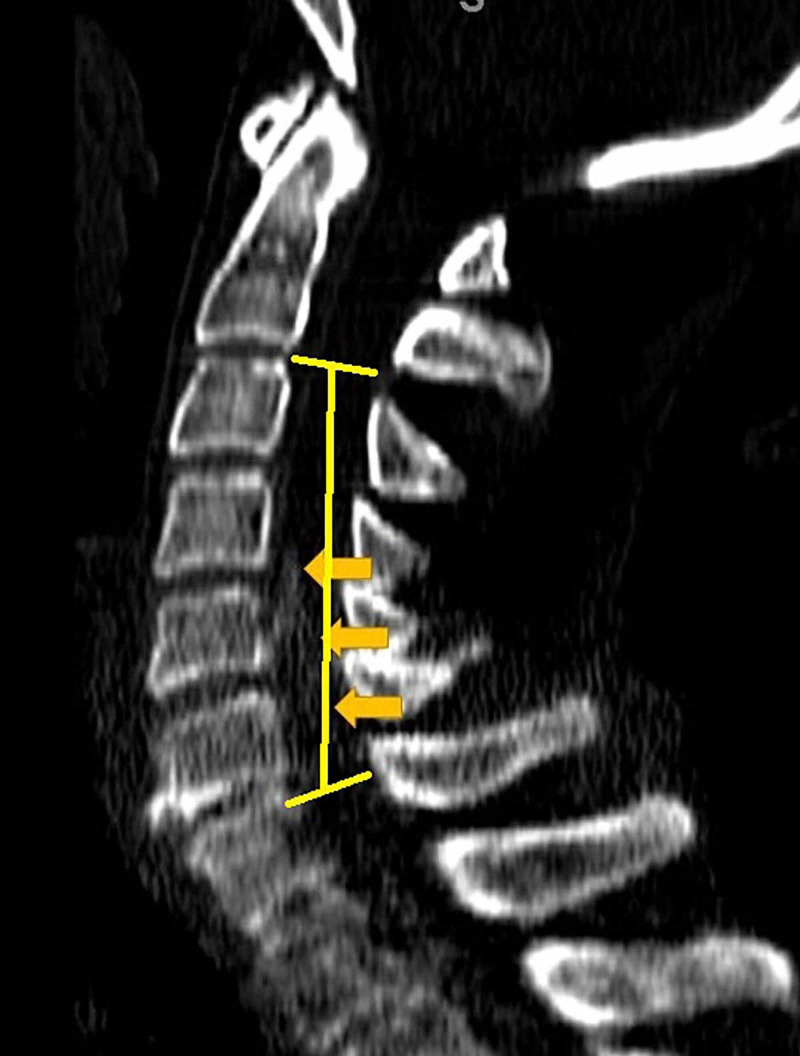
Sagittal computed tomography scan cut of the cervical spine showcasing sever ossification of the posterior longitudinal ligament (orange lines) contacting the K line drawn from C2 to C7.
